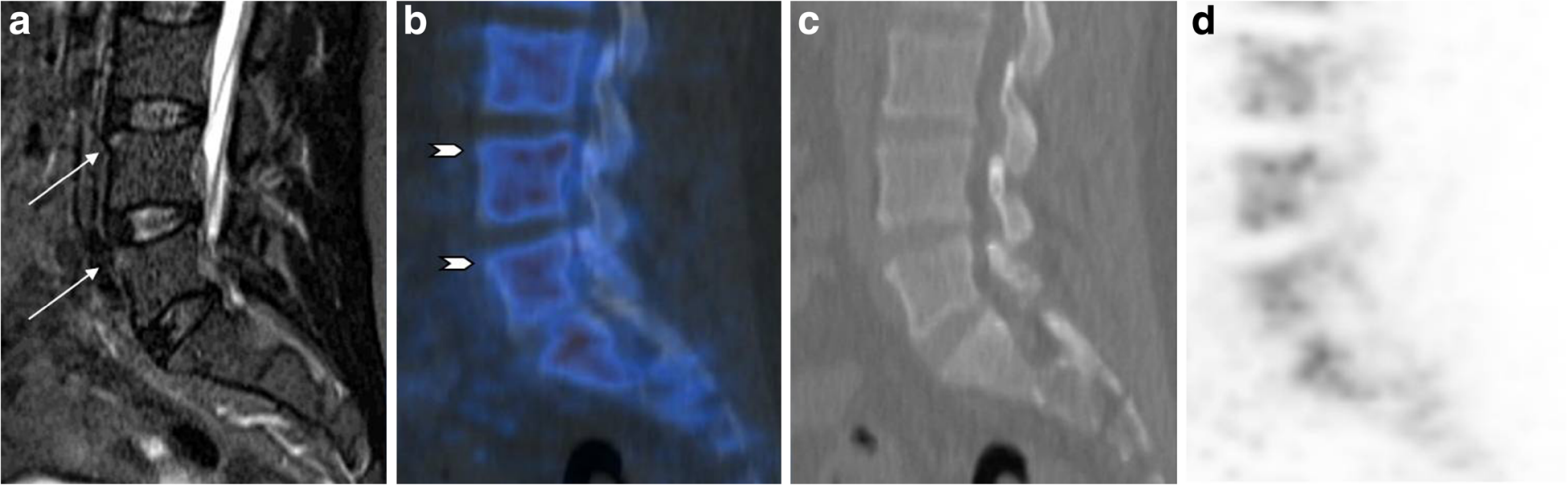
Forty-four-year-old male patient (patient number 9). Patient with an u-aCIL L4, L5 on MRI (arrows) without corresponding increased activity on F PET/CT (arrowheads). Sagittal STIR (a), fused PET/CT (b), CT (c), and PET (d) images.
